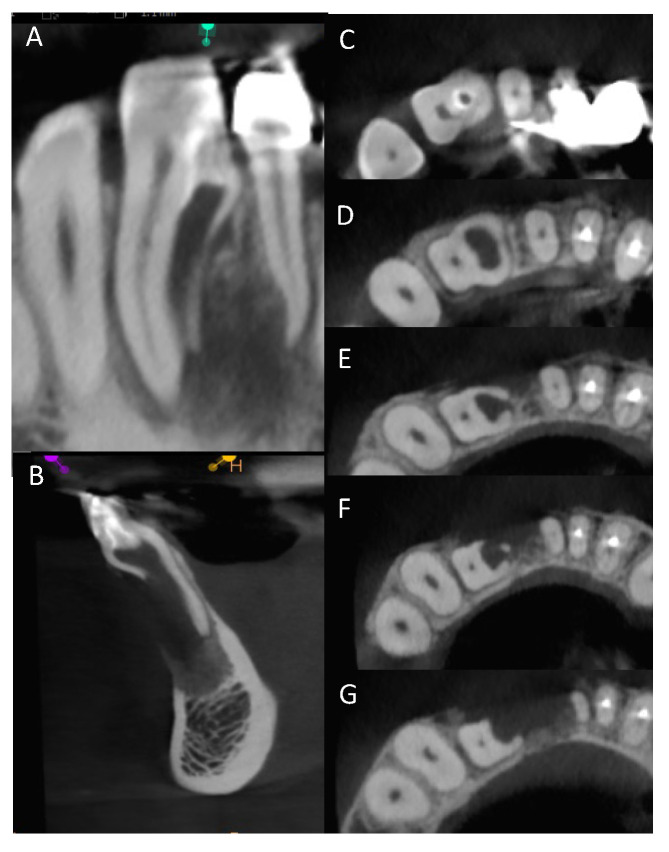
(A) CBCT coronal section of tooth #26. (B) CBCT sagittal section. (C) Coronal axial section with both chamber spaces. (D) Axial section apically within the coronal third showing dilated space of DI. (E) Axial section midroot area with lateral DI foramen to the mesial. (F) Axial section in apical third showing the mesiofacial projection and the lingual wing. (G) Apical aspect of DI with lingual wing formation.
